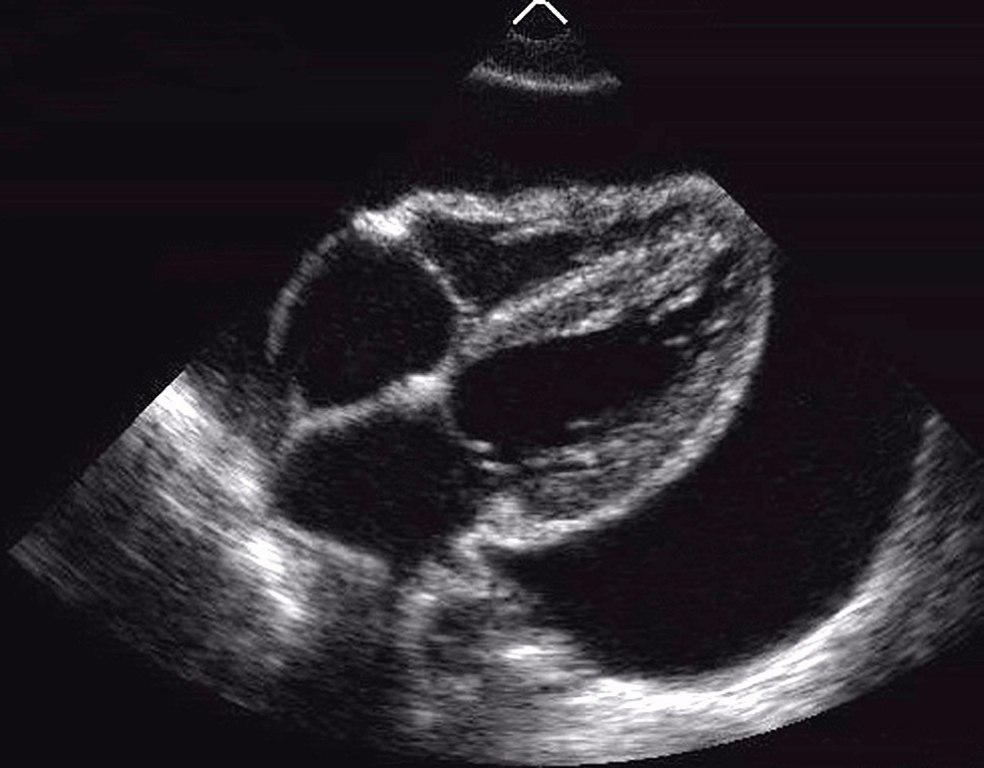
Point of care ultrasound showing pericardial effusion
